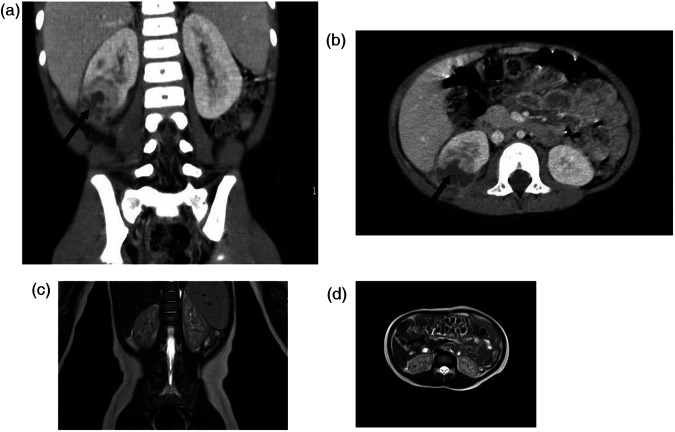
The changes of CT before treatment (a,b), and MRU after about 1 month of treatment (c,d) in case five. (a,b) The abscesses in lower pole of the right kidney were measured as about 2.4 × 2.7 cm (arrow), (c,d) The abscesses was largely disappeared.
